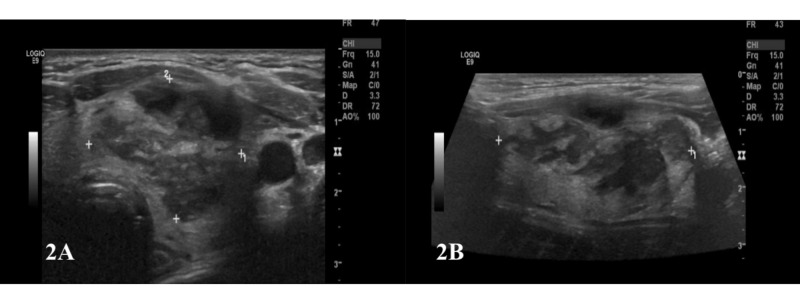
Thyroid ultrasound Images A and B showing an ill-defined 2.7 x 2 x 4.3 cm heterogeneous mass occupying most of the left lobe.
